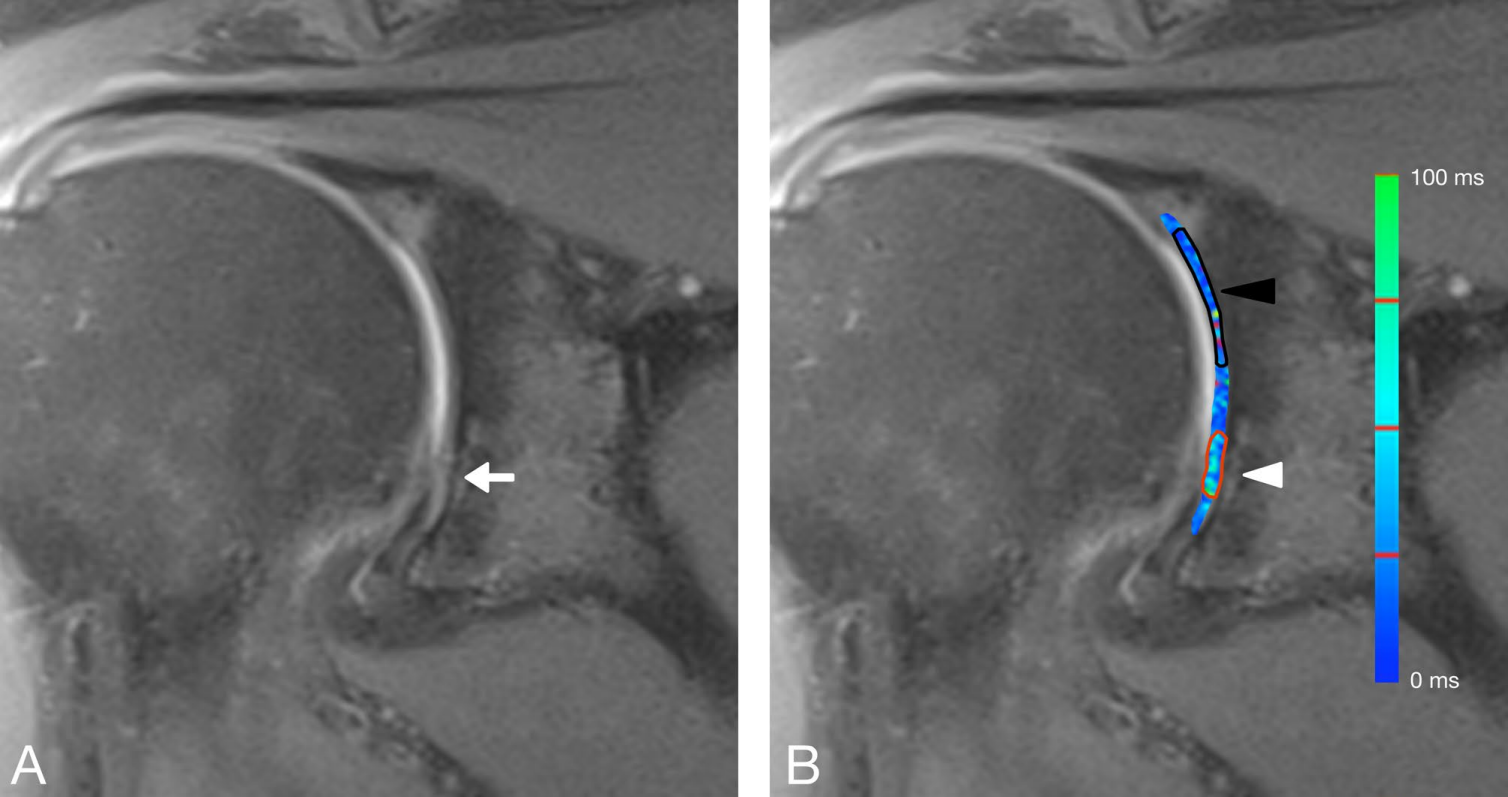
(A) shows a coronal proton-density weighted fat saturated magnetic resonance image of a 53-year-old male patient with a focal anteroinferior cartilage lesion (white arrow). (B) shows a merged image of the coronal proton density fat-saturated section and the corresponding color-coded T2 map. The average T2 mapping value in the area of the focal cartilage defect (white arrowhead, red ROI) was 45.67 ms (Reader 1, Read 1) whereas that for the normal-appearing cartilage in the anterosuperior quadrant (black arrowhead, black ROI) was 22.7 ms (Reader 1, Read 1). The average T2 mapping value of normal cartilage in the anteroinferior quadrant (ROI not shown) was 20.43 ms (Reader 1, Read 1).
